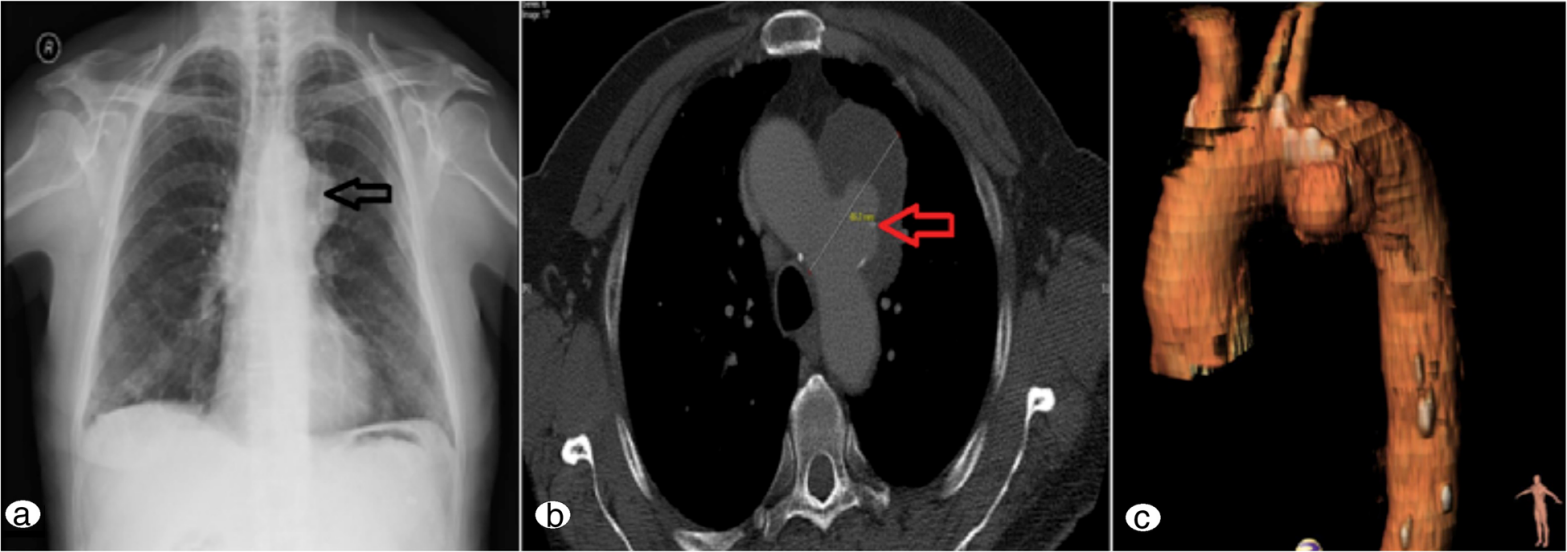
Pre-operative imaging demonstrating the pseudo-aneurysm. (a) Chest x-ray demonstrating dilatation of the aortic arch (black arrow); (b) CT angiogram (axial view) demonstrating the aortic arch pseudo-aneurysm; (c) CT angiogram showing the pseudo-aneurysm located in the medial part of the aortic arch.
